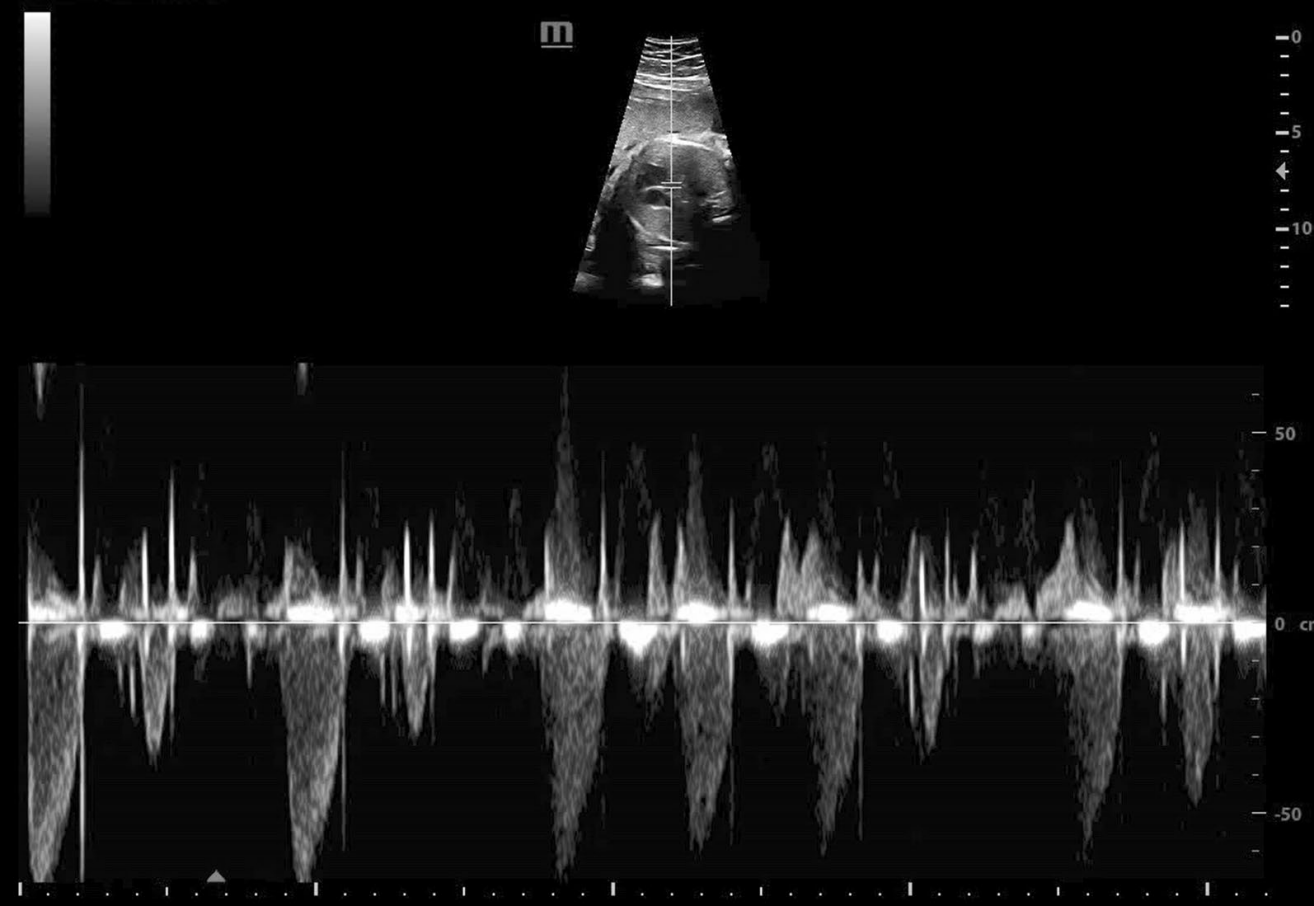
The pulsed Doppler sample gate was placed to include the outflow and inflow of the left ventricle. It shows the occurrence of frequent premature ventricular contractions (PVCs) with the full compensatory pauses and the first part being in a bigeminy pattern
